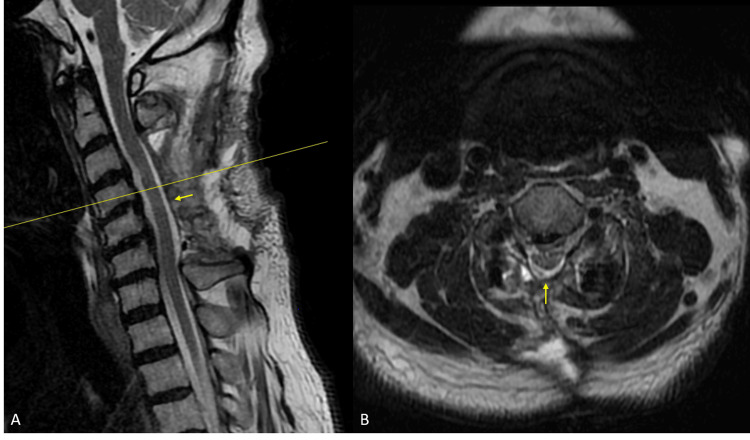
Postoperative sagittal (A) and axial (B) MRI demonstrating the re-expansion of the cervical thecal sac (yellow arrows) after drainage of the seroma
